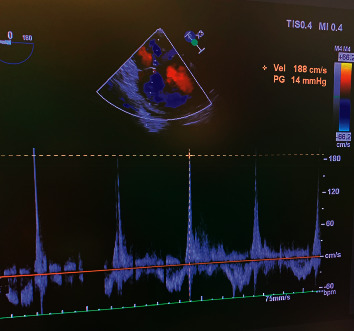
Doppler across the tricuspid valve demonstrating a peak gradient of 14 mmHg.
